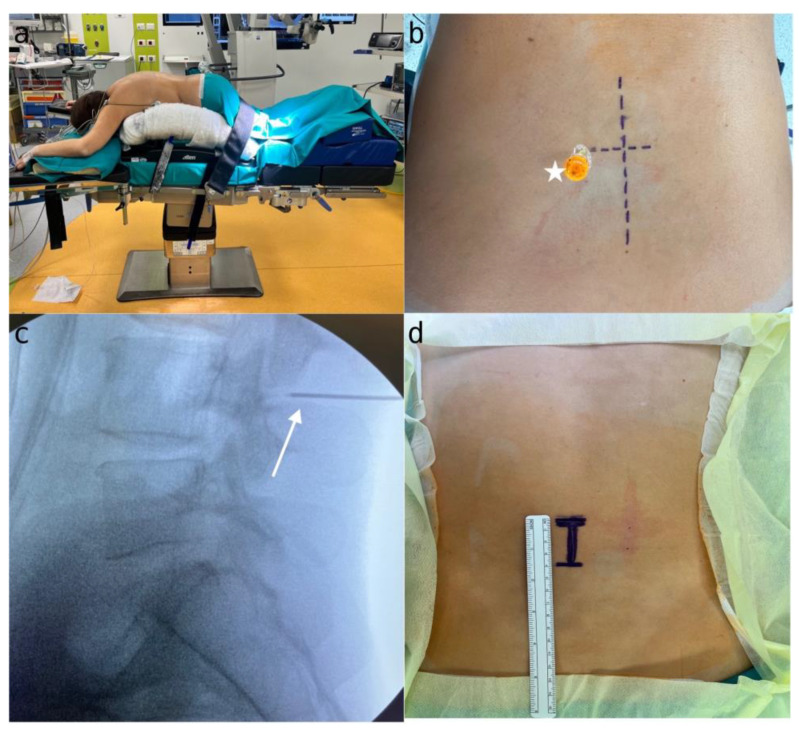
(a) Patient positioning on the Wilson frame and setup of the operating room; (b) spinal needle (white star) placed laterally on the opposite articular process of the surgical site, with the long line indicating the midline and the short line marking the L4L5 level; (c) X-ray confirmation of spinal needle placement (white arrow) to ensure correct targeting of the L4 level; (d) skin incision marked, with one-third extending superiorly and two-thirds inferiorly from the point of interest, measuring approximately 4 cm in length.
